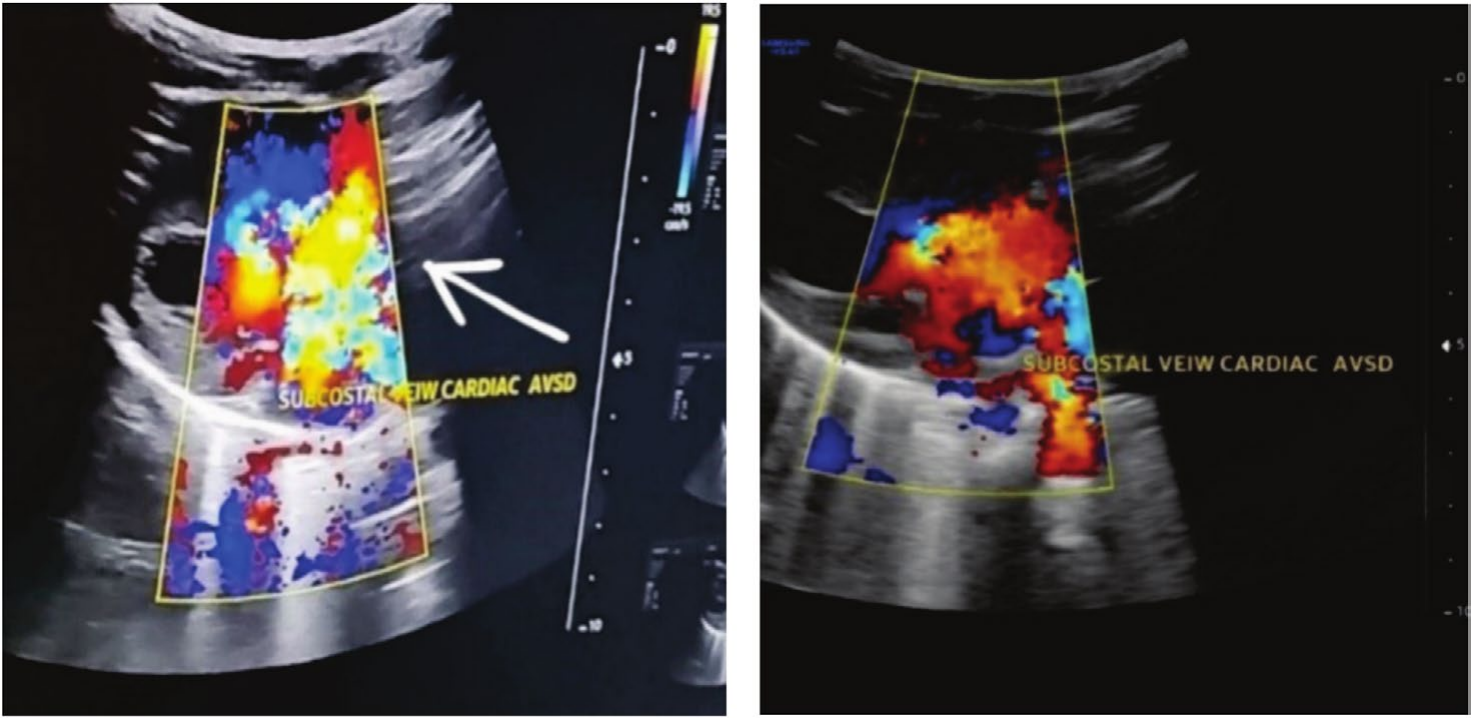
Echocardiography on subcostal view of color Doppler imaging showing a direct mixing of blood on both mitral and tricuspid valves (indicated by a white arrow), along with a 1.5‐cm‐wide open atrioventricular septal defect.
